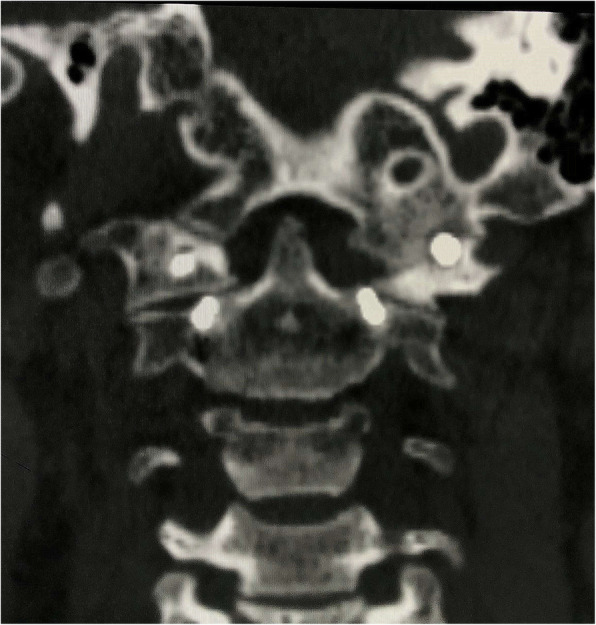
Bony fusion of the left atlantooccipital joint was confirmed by CT scan at 6 months postoperativ
